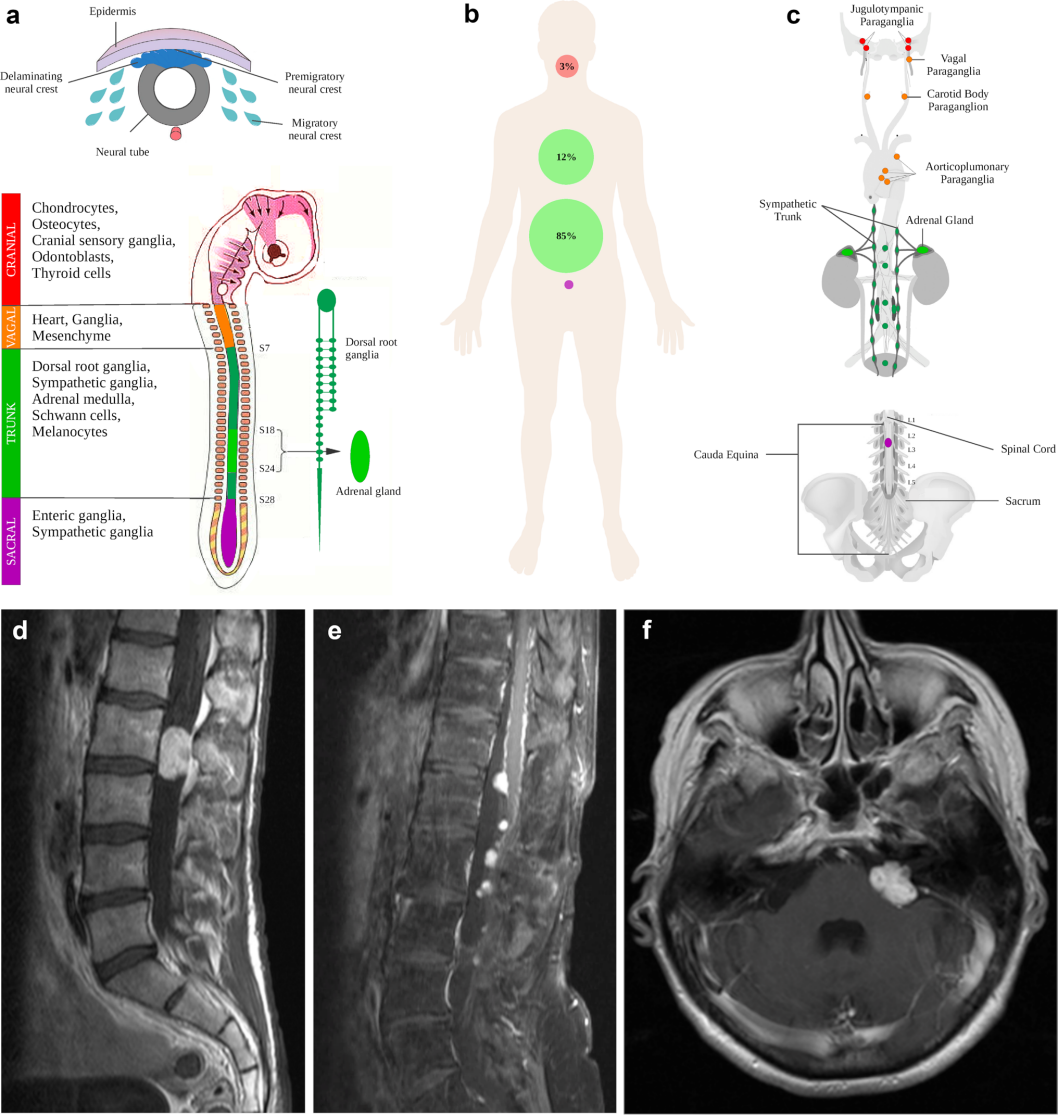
Developmental, clinical and radiological characteristics of paragangliomas. a Paraganglia develop from neural crest cell precursor cells that migrate to various body sites. Different subpopulations are recognized that differ in their spatial predominance and capability of giving rise to functional or non-functional paragangliomas. b Distribution and frequency of paragangliomas in the human body: cauda equina paragangliomas are exceptionally rare. c Common sites of paraganglioma origin in detail with type of precursor neural crest cell population indicated by color. d Typical MRI presentation of a paraganglioma in the cauda equina region as a circumscribed, oval-shaped, contrast-enhancing mass at L2 on a sagittal T1 image with contrast agent. e Paraganglioma with unusual dissemination up to the thoracic spine upon tumor recurrence (sagittal T1 image with contrast agent). More often, dissemination occurs within cauda equina nerve roots in the lumbar compartment. f Same patients as in (e) with a paraganglioma metastasis in the cerebellopontine angle—mimicking a glomus jugulare tumor (axial T1 image with contrast agent)
